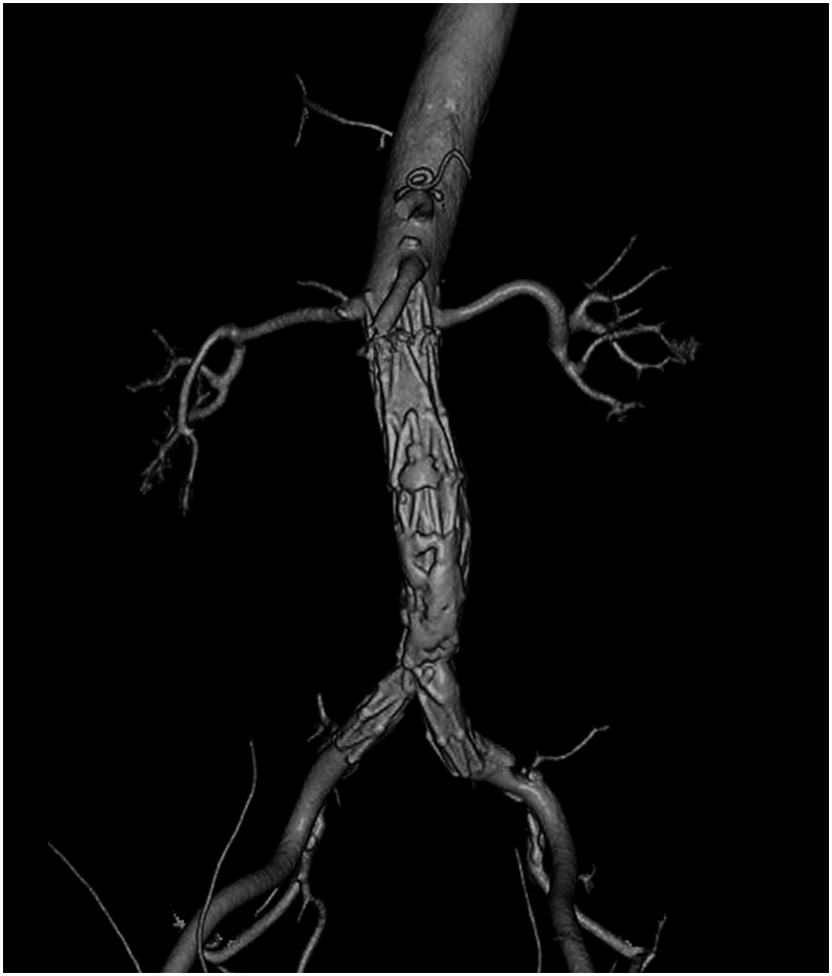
Fig. 3 Postoperative 3-dimensional computed tomography scan showing complete sealing the rupture point with no evidence of endoleak.
